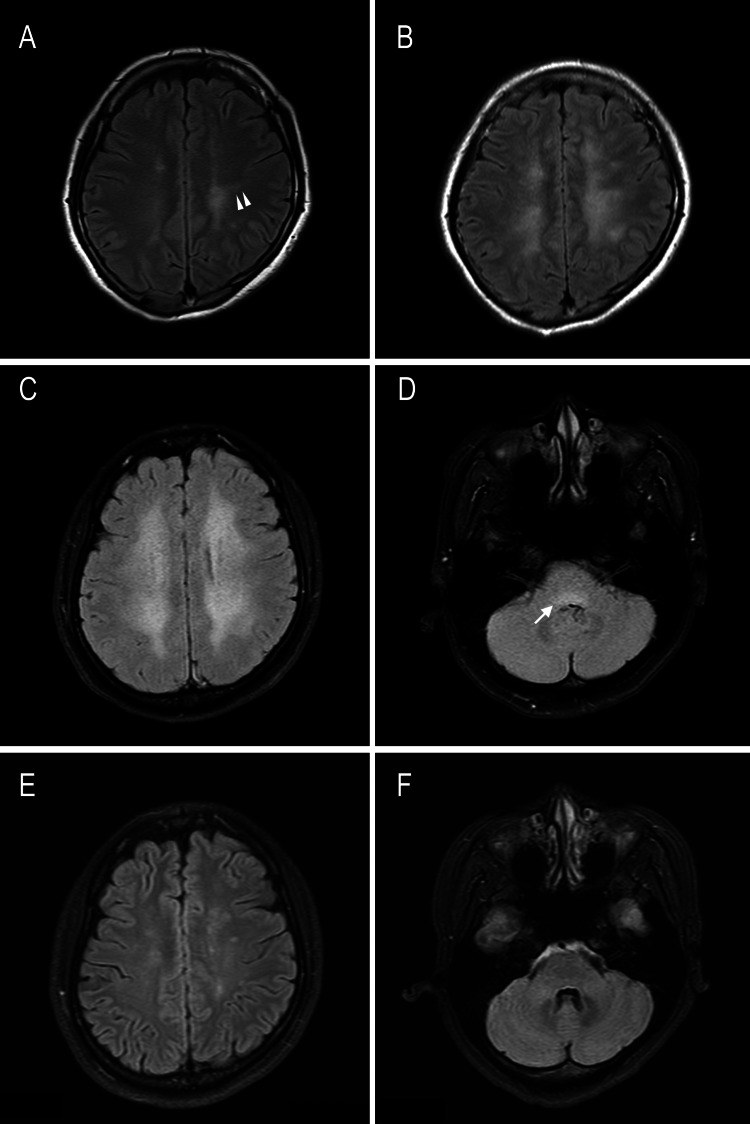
Findings of serial plain fluid-attenuated inversion recovery (FLAIR) MRI of the brain throughout treatment (A) Brain image obtained using MRI 30 days after onset showing high-intensity signals in the periventricular white matter on FLAIR images that appear as radiating and linear hyperintense lesions (arrowheads). (B) Another image obtained using MRI 30 days after onset revealing enlargement of the lesions surrounding the cerebral ventricles. (C, D) Brain images obtained using MRI at admission and 130 days after onset demonstrating further expansion of the white matter lesions and new hyperintense areas in the dorsal brainstem (arrow). (E, F) Images obtained using MRI after treatment and 150 days after onset showing reduced periventricular white matter lesions and resolution of the dorsal brainstem lesions.
